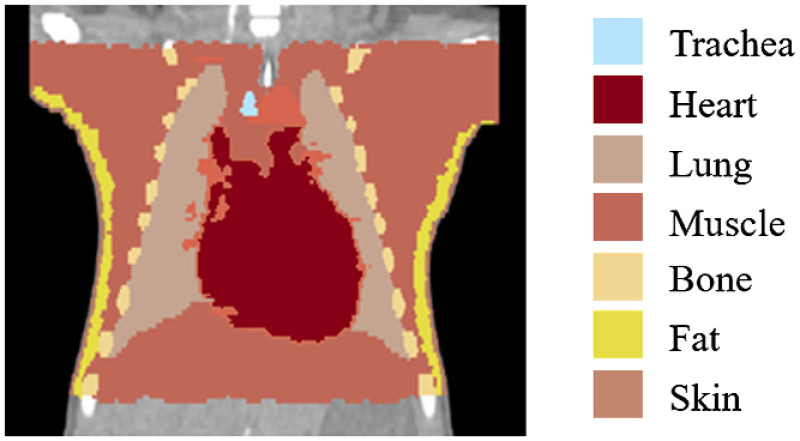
Segmentation of a DICOM image from a pseudoanonymized CT scan of a neonate, where the main organs (lungs, heart, muscle, bone, fat, and skin) have been identified and color coded.
